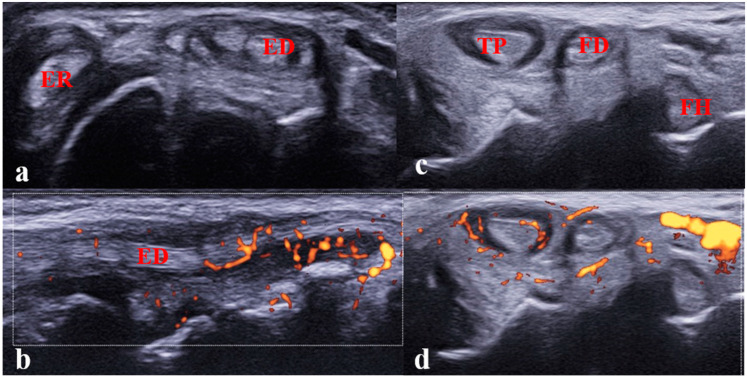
(a,b). Tenosynovitis of the extensor digitorum and extensor carpi radialis tendons in a 12-year-old girl with JIA. (a) Transverse scan showing tenosynovitis on B-mode; (b) longitudinal scan showing abnormal vascularization on power Doppler mode. Performing the longitudinal scan (B-mode/Doppler mode) is mandatory to confirm the diagnosis of tenosynovitis. ED: extensor digitorum tendon; ER: extensor carpi radialis tendon; (c,d). Tenosynovitis of the tibialis poster tendon in an 8-year-old girl with JIA. Transverse scan of the medial compartment showing tenosynovitis.
